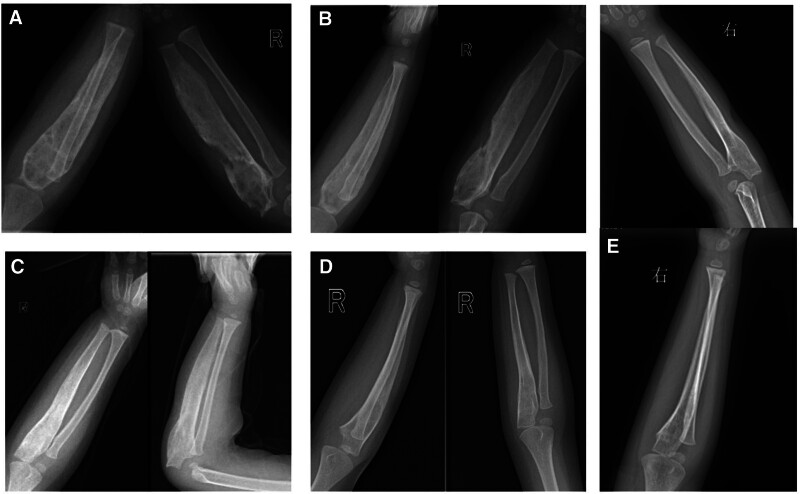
(A) At 2 years and 2 months old, X-ray imaging revealed extensive bone expansion in the ulna, with thinned cortical bone and disorganized trabecular structure. (B) At 2 years and 3 months old, X-ray indicated the ulna remained expanded with thin cortical bone, yet there was an increase in bone density. (C) At 2 years and 6 months old, X-ray imaging demonstrated a significant reduction in ulnar expansion, thickening of the cortical bone, and enhanced bone density. (D) At 2 years and 10 months old, X-ray showed the ulna’s expansion had resolved, with the bone’s shape nearing normal, normal cortical bone, and normal bone mineral density. (E) At 3 years and 6 months old, X-ray imaging confirmed normal ulnar morphology and bone structure, with no signs of recurrent bone tuberculosis or bone destruction.
